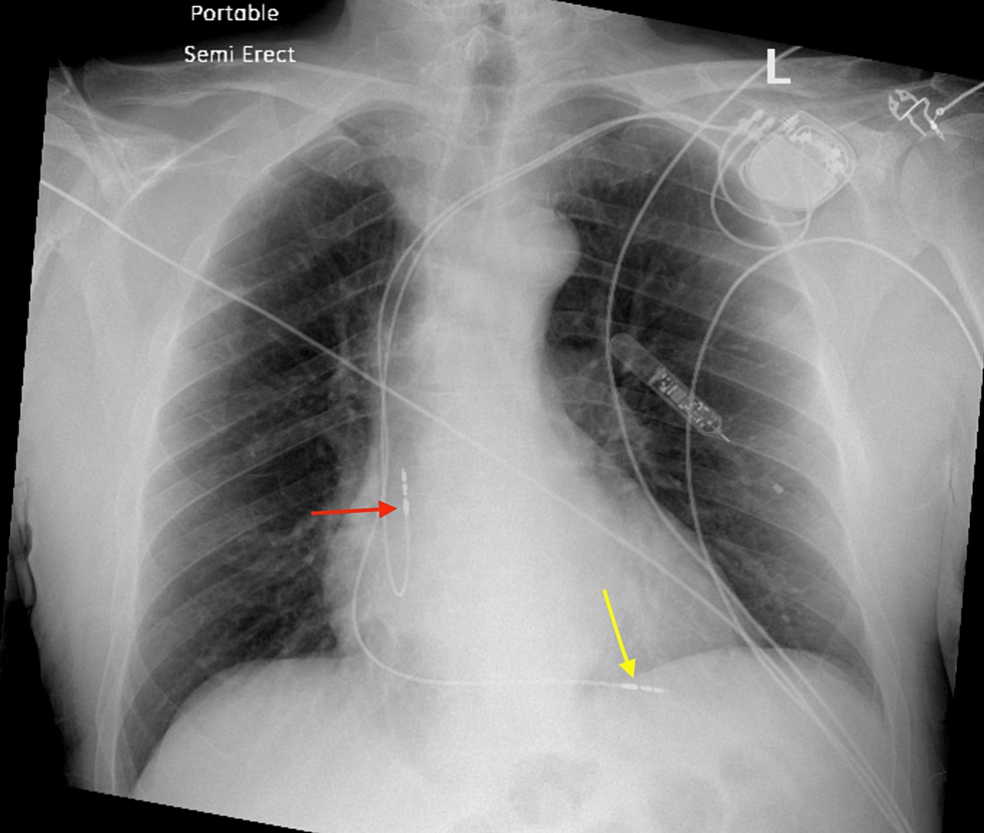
Chest radiograph (CXR) PA view Chest X-ray PA view after pacemaker insertion shows pacemaker leads in the right atrium (red arrow) and right ventricle (yellow Arrow).
